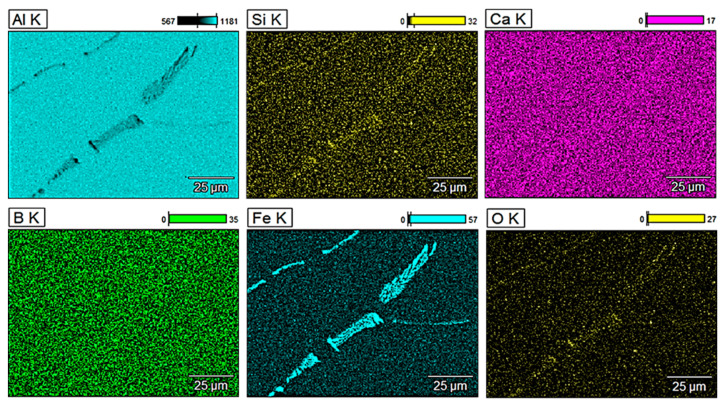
Energy-dispersive X-ray (EDX) mapping of EN AW 1370 (metallurgical synthesis).
